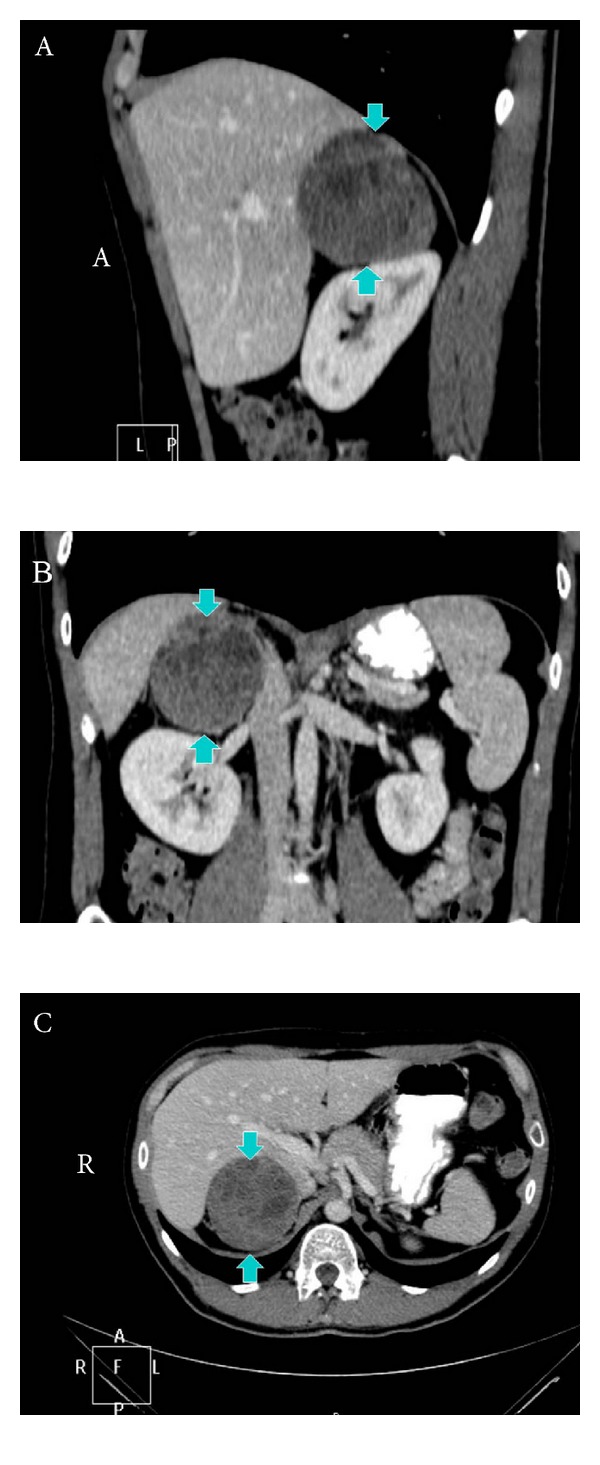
Computed tomography of the abdomen showing the right adrenal mass with malignant features, such as heterogeneous contrast enhancement. (A) sagittal plane, (B) coronal plane, and (C) axial plane (blue arrows point to the tumor).
